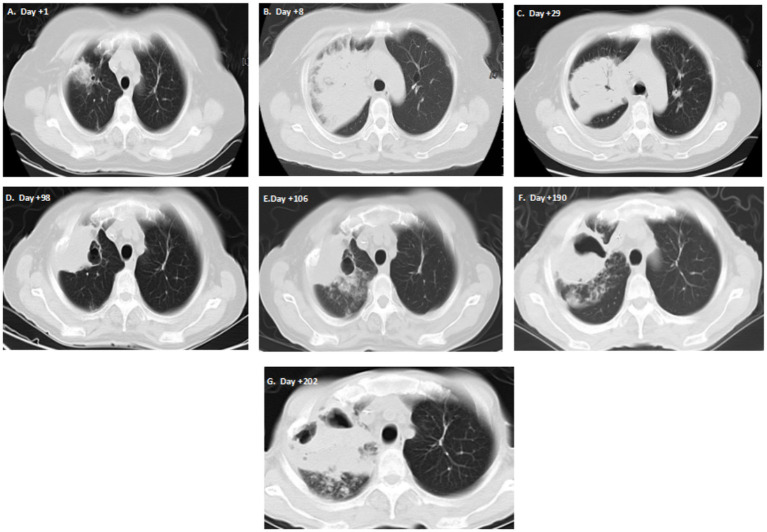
Serial chest CT images show pulmonary infection progression. (A) Day +1: Mass-like consolidation in the right upper lobe. (B) Day +8: Significant lesion progression. (C) Day +29: Cavitation development. (D) Day +98: Slight cavity enlargement. (E) Day +106: Lesion expansion during septic shock. (F) Day +190: Further progression with multidrug-resistant infection. (G) Final scan: Extensive consolidation.
